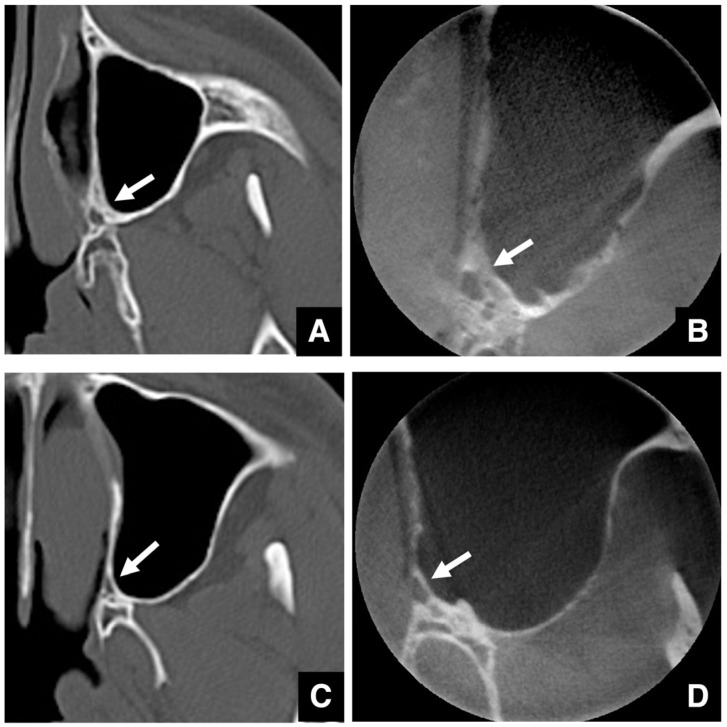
Axial images of the greater palatine canal obtained using multidetector computed tomography (MDCT; slice thickness, 3.0 mm) and dental cone-beam computed tomography (CBCT; slice thickness, 0.6 mm). (A) Axial bone window MDCT image of a patient with a thick-walled greater palatine canal (arrow). (B) Axial CBCT image of the same anatomical feature showing a thick-walled greater palatine canal with clearer delineation of the canal wall (arrow). (C) Axial bone window MDCT image of a patient with a thin-walled greater palatine canal (arrow). (D) Axial CBCT image of the same anatomical feature showing a thin-walled greater palatine canal with more clearly visualized canal margins compared with MDCT (arrow). Permission has been obtained to publish all figures.
